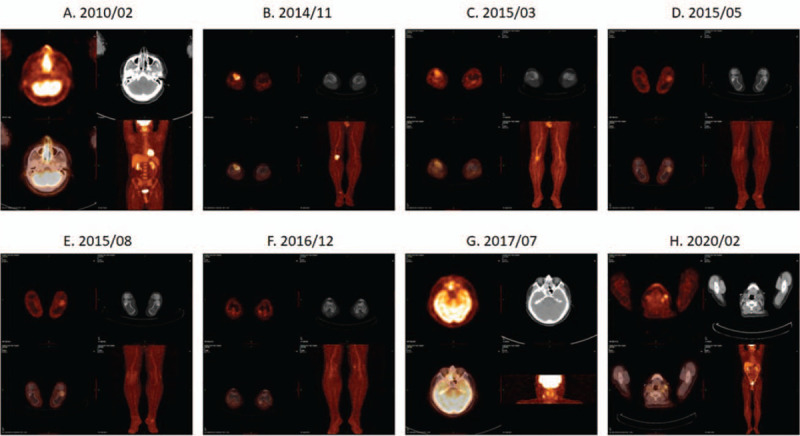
Positron emission tomography (PET) scan assessment. A. Baseline PET-CT showed increased uptakes in the nasopharynx (SUV_max_: 14.5). B. After radiotherapy and six cycles of EPOCH: Complete remission but presence of metabolic activity lesions (SUVmax: 8.9) in upper right tibiae and right calcaneum bone of right leg. C-E. After six cycles of SMILE /P-GEMOX and radiotherapy, he achieved remission. F,G. However, PET-CT imaging showed mild increase of local metabolic activity in the right lateral malleolus, the third and fourth left toe bones and the fifth right toe bone. H. Patient remains in remission as of February 2020 following treatment with Sintilimab and chidamide. PET-CT shows no evidence of relapse or metastasis. SMILE = steroid (dexamethasone), methotrexate, ifosfamide, L-asparaginase, and etoposide, SUV = standard unit value.
